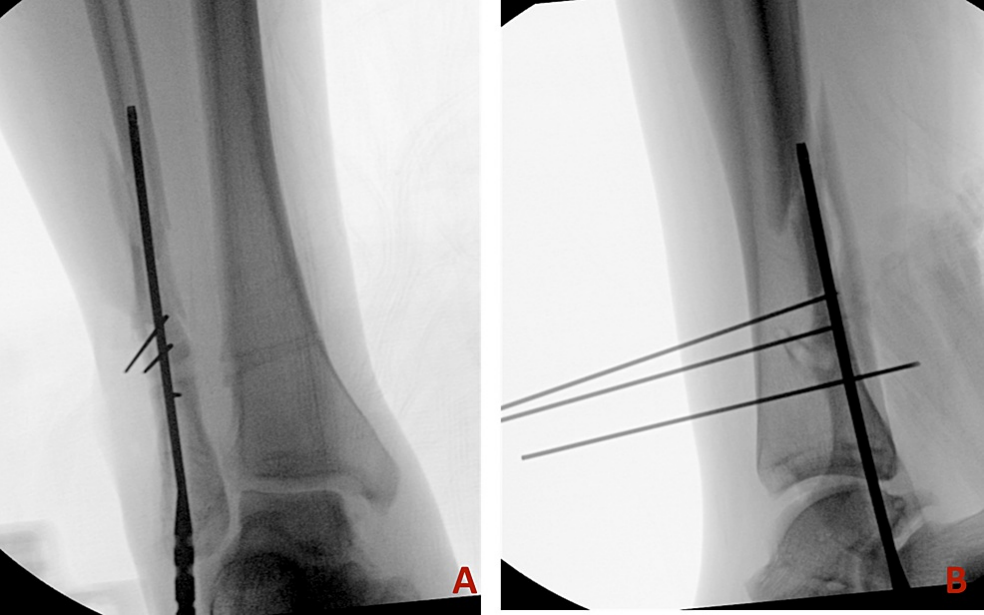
Intraoperative radiographs depicting the advancement of the fibular nail over the guidewire past the blocking wires. A: Anterior-posterior view. B: Lateral view
